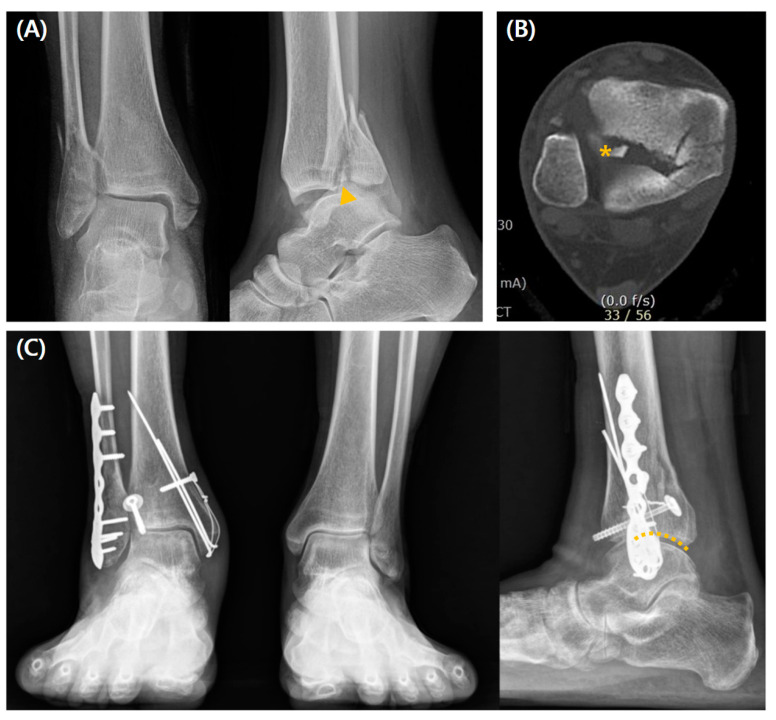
A case of a 44-year-old woman who underwent a direct approach and posterior screw fixation for type 2 PMF. (A) Preoperative radiographs show comminuted fracture (arrow), and (B) preoperative CT scan images show a posterior malleolar fragment representing type 2 fracture with the ICF (asterisk) and step-off. (C) Weight-bearing radiographs obtained 3 years postoperatively show good reduction without the development of PTOA (dotted line; anatomically reduced joint surface).
